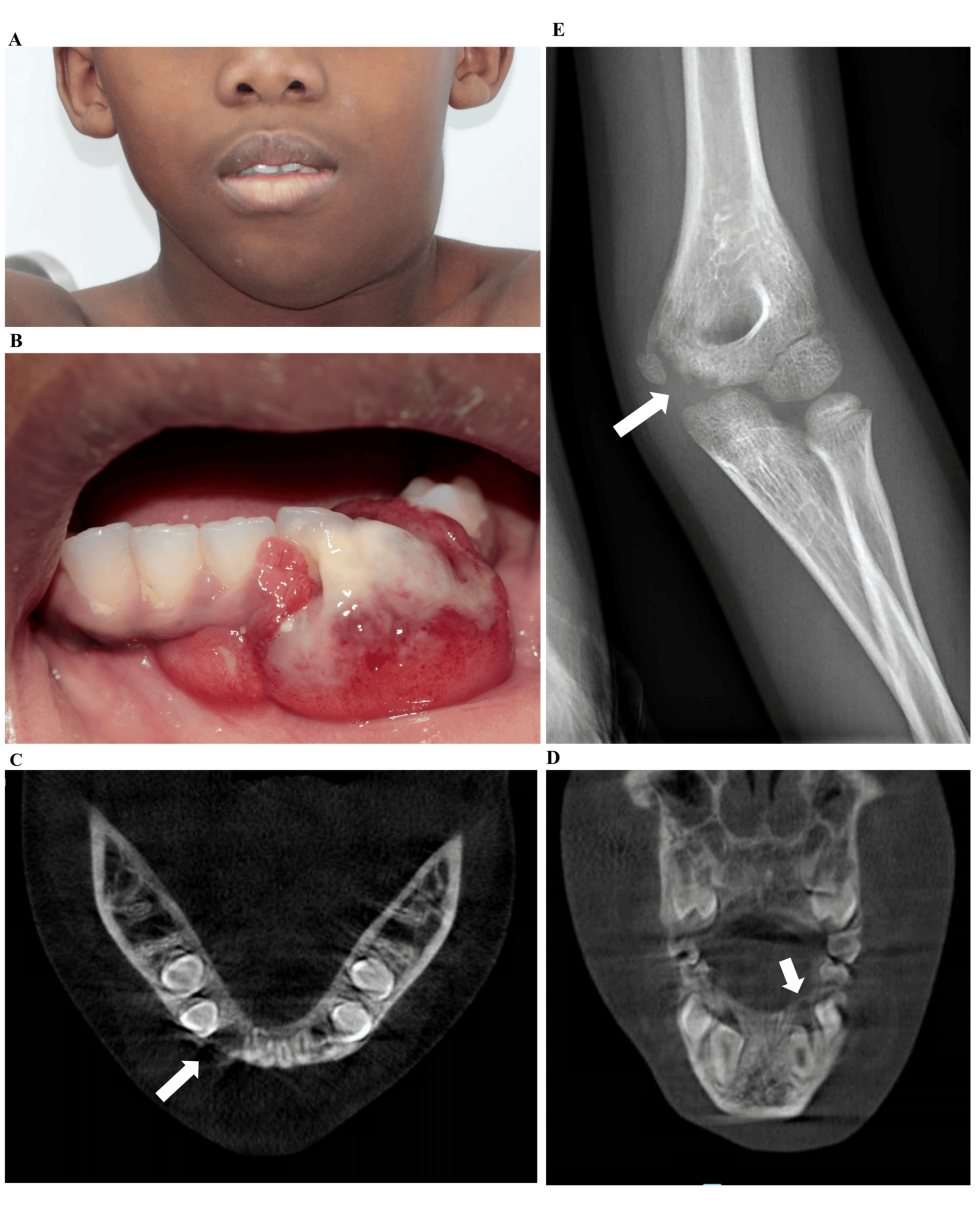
Clinical and radiographic features of primary mandibular ALK-positive anaplastic large cell lymphoma. (A) Extraoral examination showing a left cervico-mandibular swelling. (B) Intraoral examination showing a reddish, ulcerated, necrotic mass on the lower left gingiva and alveolar ridge. (C) Cone Beam Computed Tomography (CBCT), axial section, and (D) coronal section showing discrete bone resorption of the alveolar bone (arrows). (E) Radiograph of the left arm showing osteolysis of the cortical bone of the humeral epiphysis (arrow). ALK: Anaplastic lymphoma kinase.
